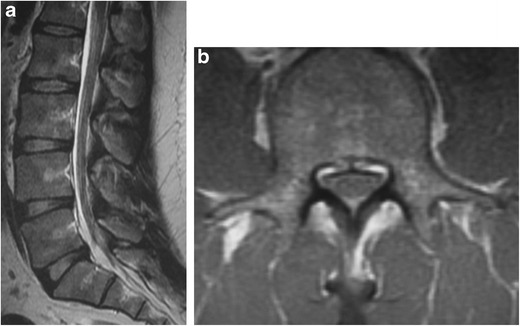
Congenital stenosis of the spinal canal: Sagittal (*a*) and axial (*b*) T1-weighted MR images of the lumbar spine reveal narrowed anteroposterior diameter of the spinal canal (< 10 mm)
